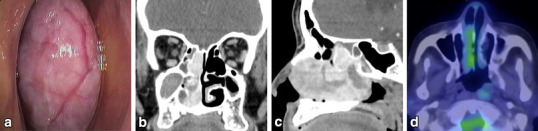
Nasal endoscopic examination showed a reddish tumor in the right nasal cavity (a). CT scan revealed a mass occupying the right nasal cavity, with strong enhancement (b, c). FDG-PET/CT showed slight uptake by the tumor (d)
